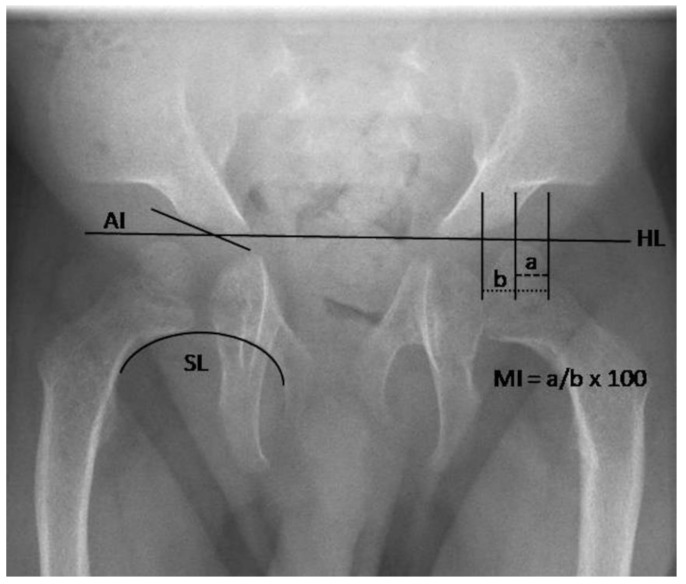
Quantitative radiographic assessments in MLII. Plain radiograph of a 2.8-year-old male patient. AI, acetabular index; SL, Shenton’s line; MI, Reimer’s migration index; HL, Hilgenreiner’s line.
